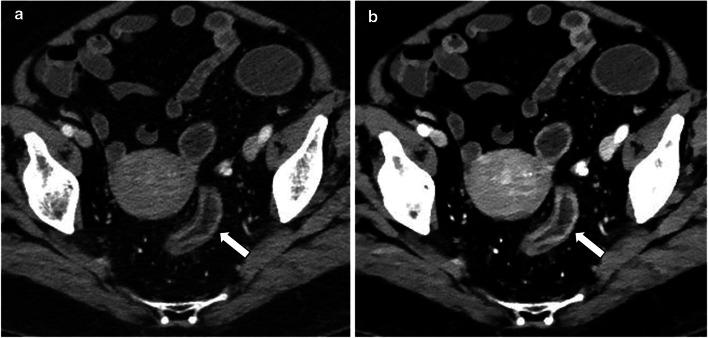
CT enterography of a 74-year-old woman with known Crohn's disease of the colon. Conventional images show mild mucosal hyperenhancement in the sigmoid colon (a) which is more conspicuous using 50 keV mono-energetic images (b)
